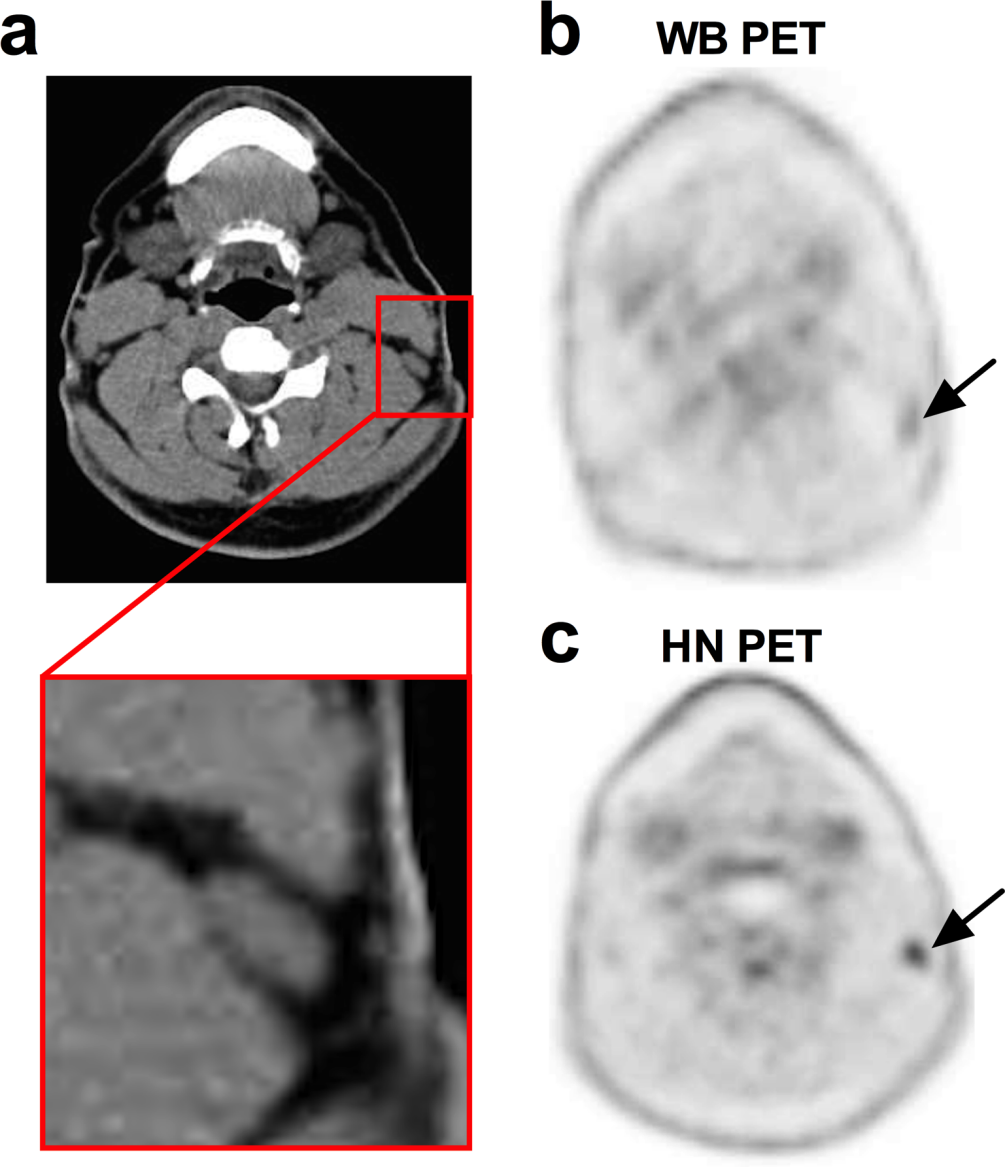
PET/CT study in a 43-yr-old patient with papillary thyroid cancer and central nodal disease. He presented a detectable rhTSH-stimulated serum Tg level (4 ng/ml) three years after initial surgery. The neck US and diagnostic ^131^I whole-body (WB) scan were negative. Hybrid CT scan of the PET/CT study is presented in Panel a. On the WB PET (Panel b) there was a faint FDG uptake (SUVmax = 2.1) in a left lateral LN which was considered as probably benign (score 2) by Reader 1 and indeterminate (score 3) by Reader 2. On head and neck (HN) PET (Panel c), both readers reported a probably malignant (score 4) FDG focus (SUVmax = 3.4). Node/muscle background ratio was 4.3 on HN PET vs. 2.3 on WB PET. A LN without suspicious features (short-axis: 6 mm; long axis: 14 mm) was described in front of this FDG focus on hybrid CT scan (Panel a).
